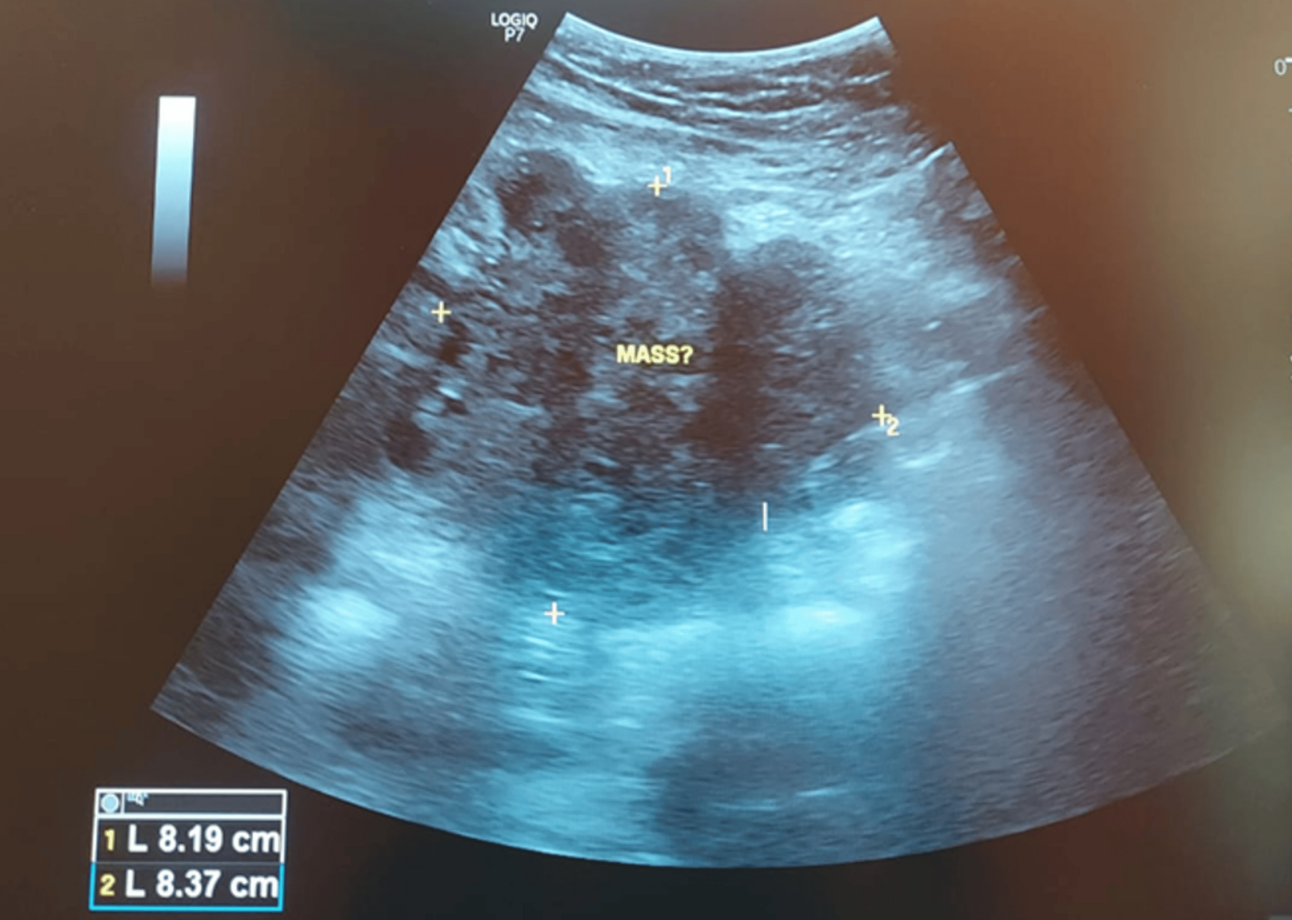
Ultrasound showing a mass on the lower left abdomen.
